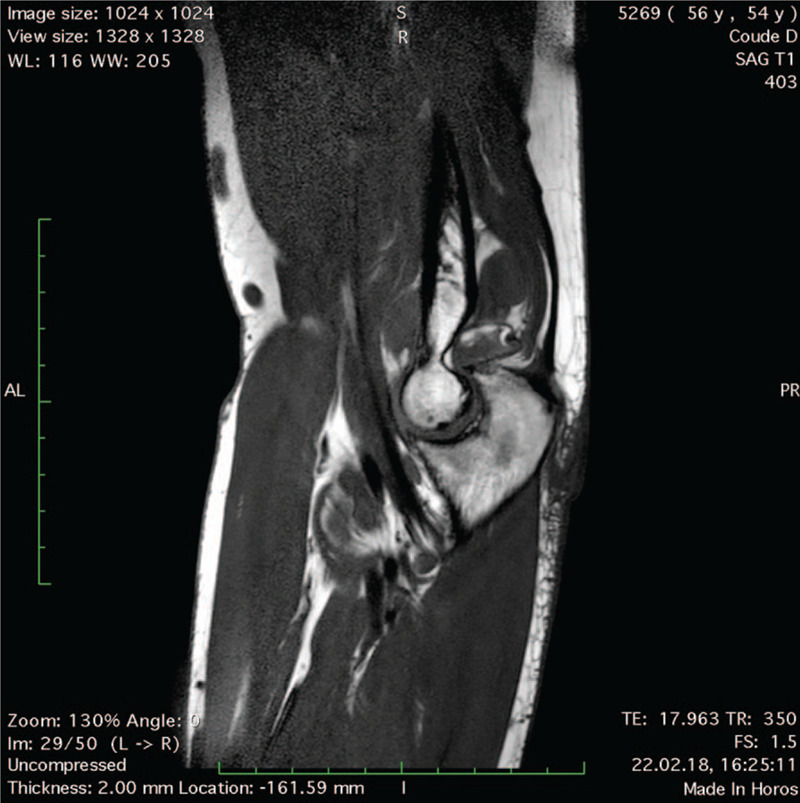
MRI T1 sequence sagittal view of the right elbow showing posterior humeroulnar impingement caused by LA.
